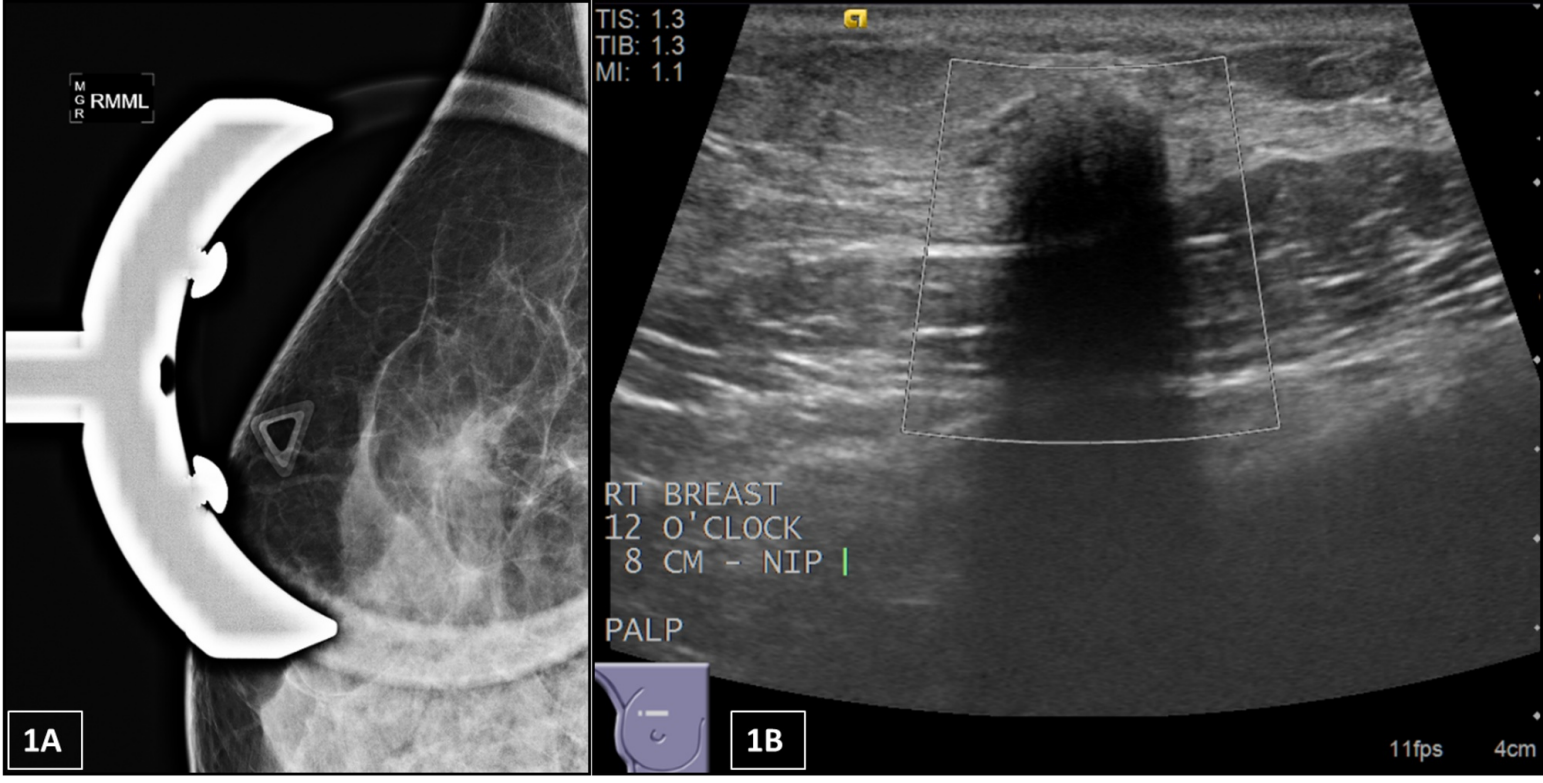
(A) Mammography of the right breast from one of the patients with a granular cell tumor. Triangle marker over the palpable area of concern; there is an irregular mass with indistinct margins. (B) Ultrasound shows an irregular hypoechoic mass with indistinct margins and posterior shadowing with no vascularity measuring 9 x 8 x 8 mm. The radiology categorized this lesion as BI-RADS Category 5 – highly suspicious for malignancy, biopsy recommended. BI-RADS: Breast Imaging Reporting and Data System
